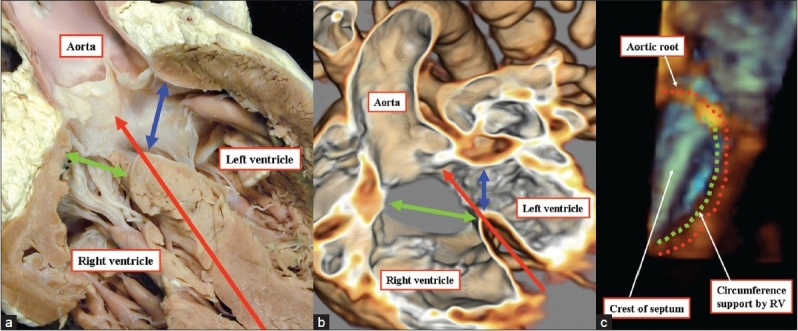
(a) The anatomic section shown to the left hand is a four-chamber cut through the overriding aortic valve in a patient with tetralogy of Fallot. The plane representing the interventricular communication is the upward continuation of the long axis of the ventricular septum (red arrow). It is the right ventricular margin of the cone of space subtended by the overriding valve (green double headed arrow) that is usually considered to represent the ventricular septal defect. The blue double-headed arrow is the effective outflow tract from the left ventricle. (b) A CT angiogram (middle panel) replicates the anatomic section. The plane representing the interventricular communication is the upward continuation of the long axis of the ventricular septum (red arrow). It is the right ventricular margin of the cone of space subtended by the overriding valve (green double headed arrow) that is usually considered to represent the ventricular septal defect. The blue double-headed arrow is the effective outflow tract from the left ventricle. (c) A three dimensional echocardiogram shown to the right hand shows that the plane of the ventricular septum truly divides the overriding aortic root into its right and left ventricular components, this plane being the interventricular communication
